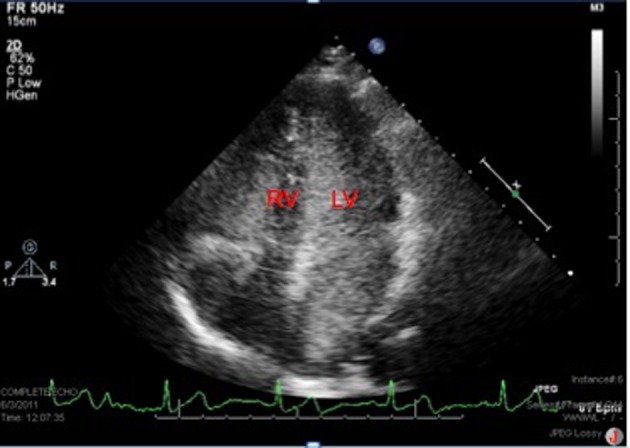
The 2 D echocardiogram showing bubbles predominantly in the left atrium and left ventricle after agitated saline injection in the left arm, note few bubbles in the right heart as well due to the ASD and left to right shunt.
